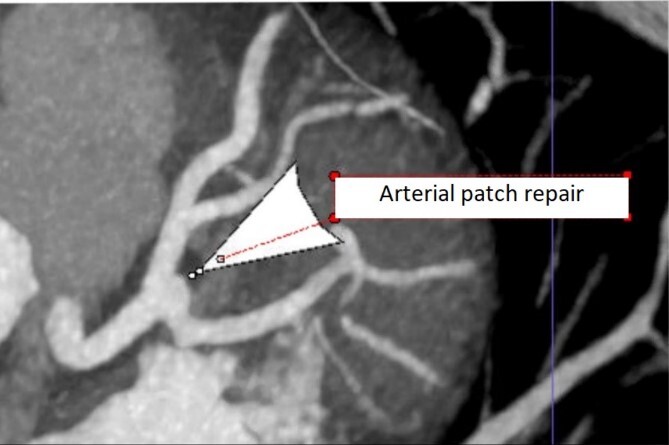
Repeat coronary CTA at 3 month follow up showing normal appearing distal LMCA.
